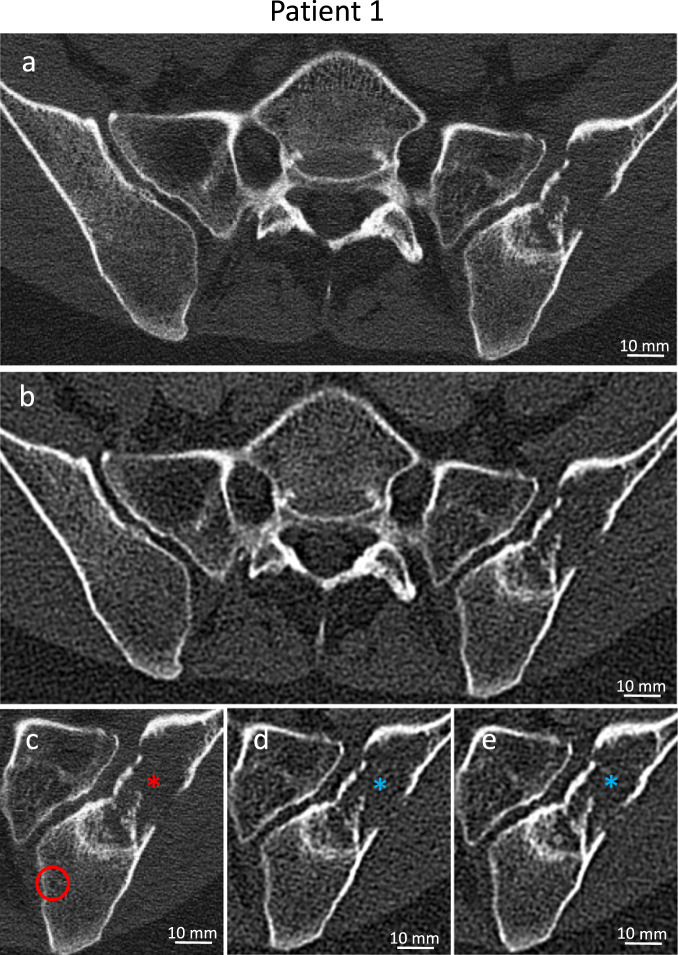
Examples of PCD CT and conventional EID CT images. a–e Unenhanced CT images of a 45-year-old patient with high-grade primary small cell carcinoma of the breast demonstrating an osteolytic lesion of the left iliac bone after radiation. a, c PCD CT images (U80f, 300 mAs, 2019/10, FOV 275 mm), b, d, e EID CT images (B80f, 124 mAs, b, d 2019/09, e 2019/12, FOV 500 mm). c, d are zoomed-in displays of a and b; c–e the small osseous defect shown in the PCD CT images (red circle) is visible to a moderate extent just in the low energy dataset (100 kV) of the dual-energy EID CT data.
